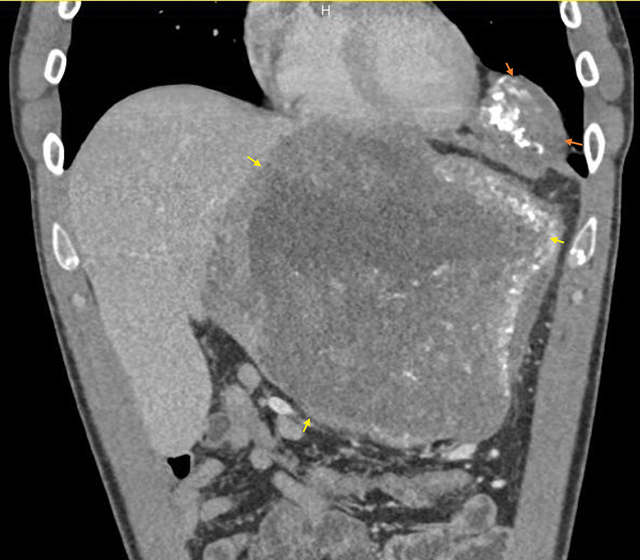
Portal CT coronal plane. Left hepatic (yellow arrows) and left cardiophrenic masses (orange arrows) with calcifications.
